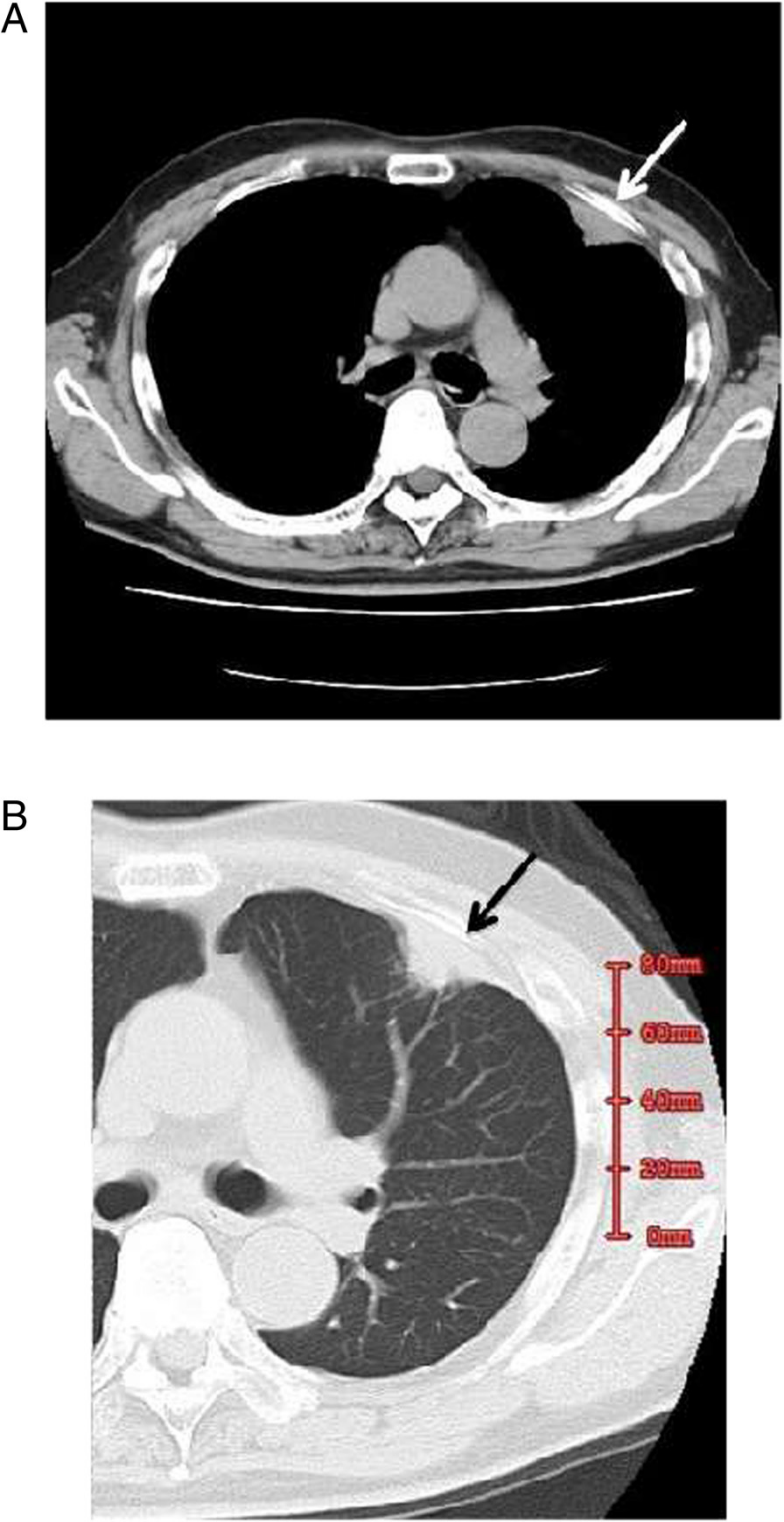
Computed tomography. (A) Pleural tumor is observed on the left chest wall. (B) Thin-slice CT shows the silhouette sign-positive tumor with an irregular surface. Arrows indicate the tumor at the pleura.
